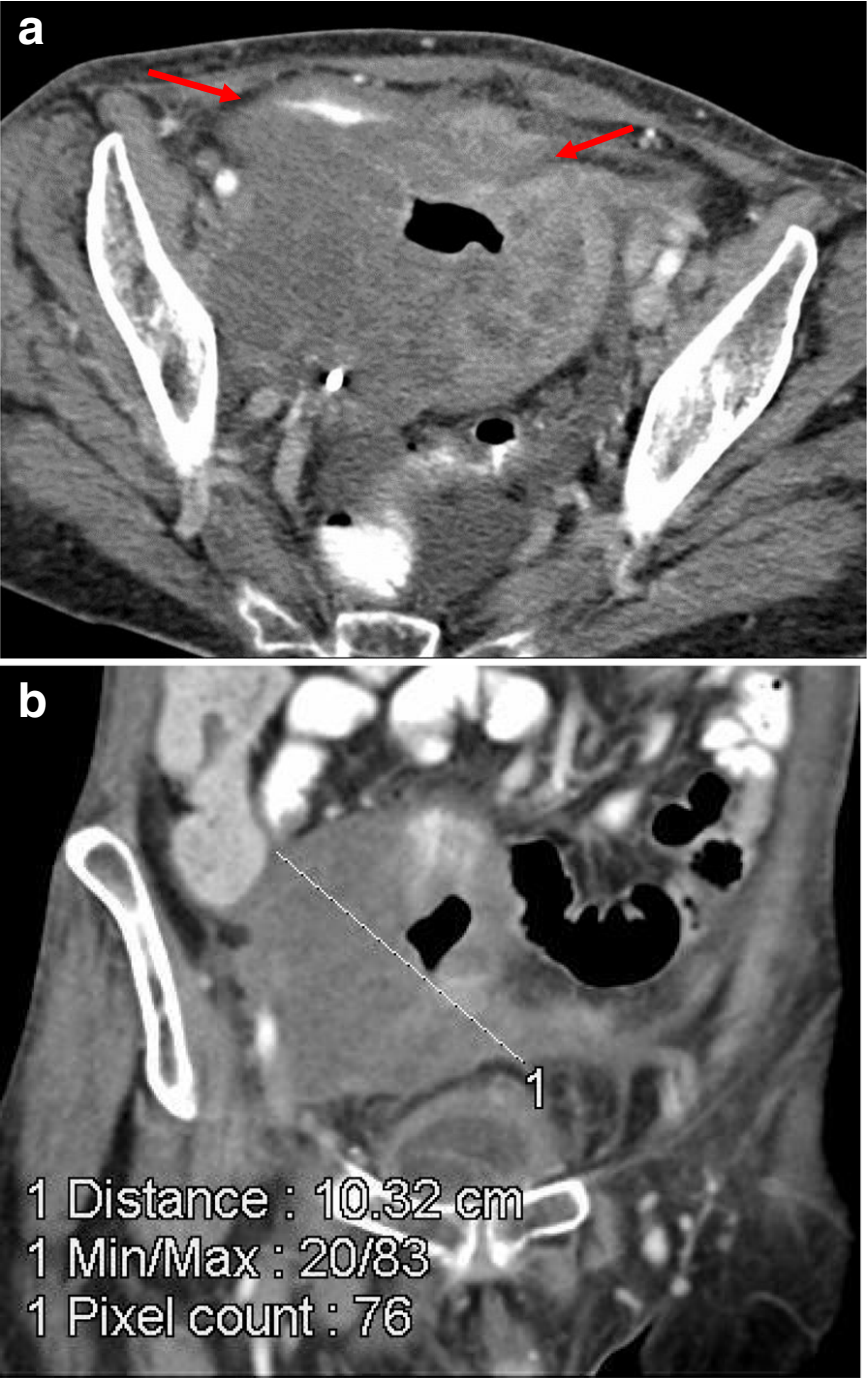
a and b Computed tomography scan showing partial response after 3 months of treatment. *Arrows* on panel a are pointing to the pelvic mass
